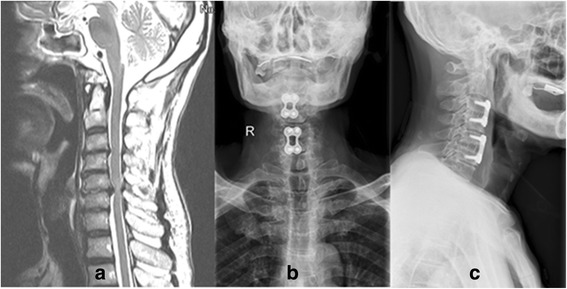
Preoperative lateral T2-weighted MRI (a) showing a 68-year-old man with C3-C4 and C5-C6 disc herniation. Anteroposterior (b) and lateral (c) radiographs showing C3-C4 and C5-C6 anterior cervical discectomy and fusions (ACDF) with cages and titanium plates
